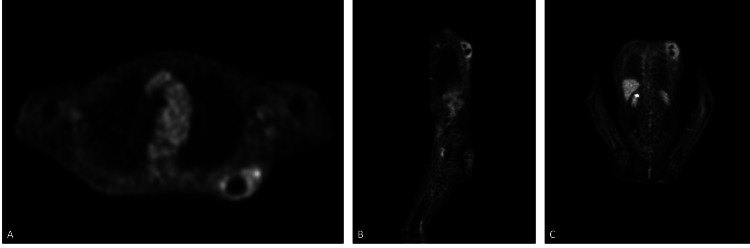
Positron emission tomography/computed tomography imaging. A: Transverse section of the F18-fluorodeoxyglucose positron emission tomography/computed tomography imaging revealing a primary tumoral mass lesion in the dorsal region accompanied by hypometabolic areas due to possible necrosis in central regions. B: Saggital section of the F18-fluorodeoxyglucose positron emission tomography/computed tomography imaging revealing a primary tumoral mass lesion in the dorsal region accompanied by hypometabolic areas due to possible necrosis in central regions. C: Coronal section of the F18-fluorodeoxyglucose positron emission tomography/computed tomography imaging revealing a primary tumoral mass lesion in the dorsal region accompanied by hypometabolic areas due to possible necrosis in central regions.
